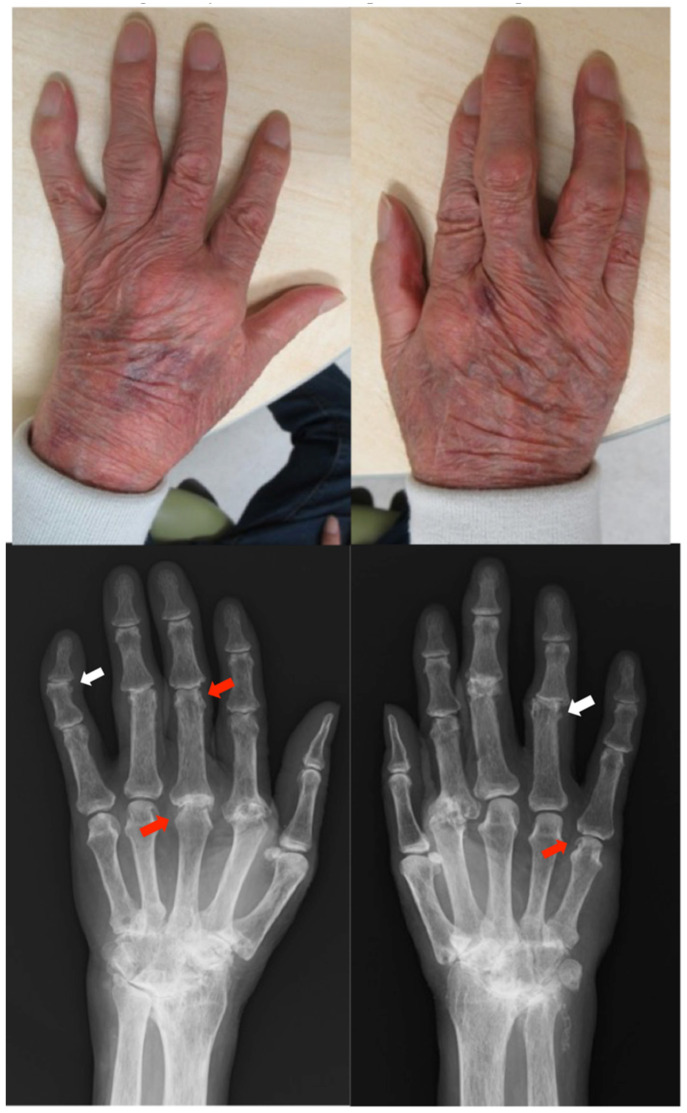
Clinical photographs and radiographs of the hands in case 1. The finger joints are swollen and deformed. Radiographs show symmetrical subluxation of the second MCP joints and bone erosions at the PIP and MCP joints (red arrow). Severe osteolysis and destruction occur on multiple joint surfaces. Severe joint space narrowing and tonic change are depicted in both carpal bones. The left DIP and right PIP joints show bone proliferation suspicious for PsA (white arrow). MCP, metacarpophalangeal; PIP, peripheral interphalangeal; DIP, distal interphalangeal.
